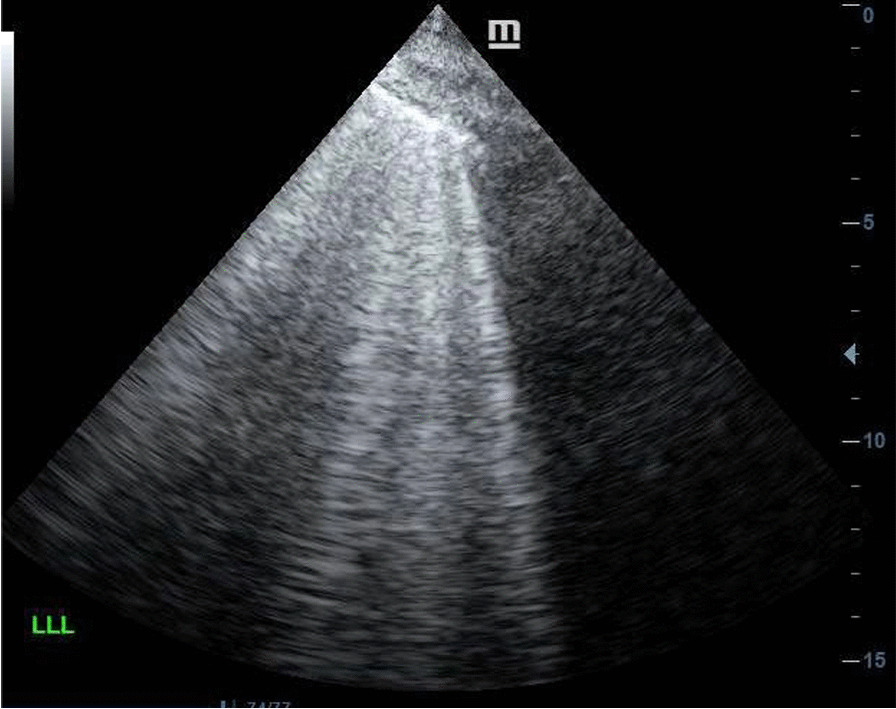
Lung ultrasound image showing the presence of multiple B-lines, the so-called “B-profile” in a patient with *P. jirovecii*
